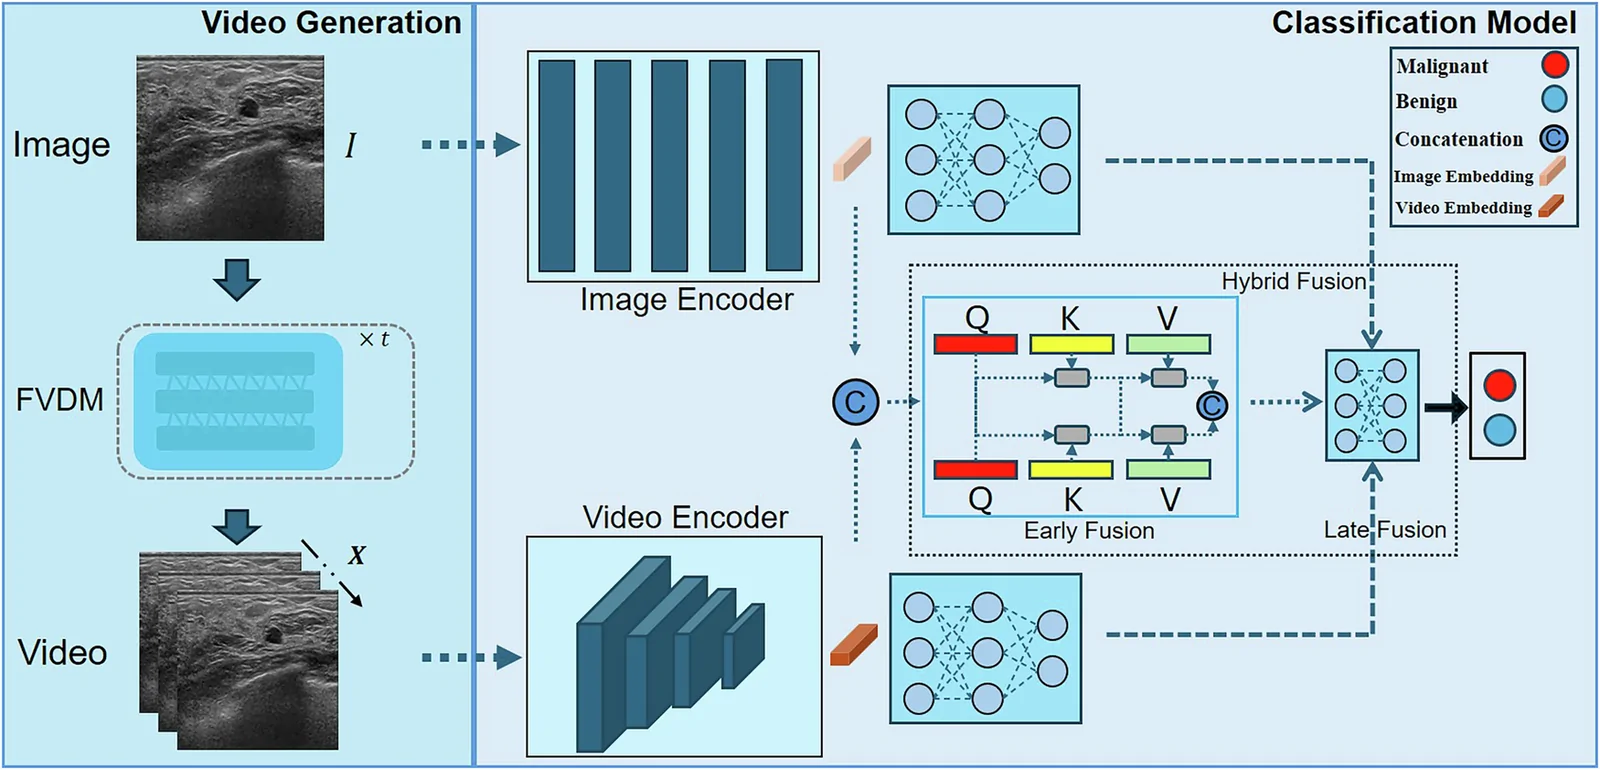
The proposed breast ultrasound classification framework consists of two stages. In the first stage, a diffusion-based model generates a breast ultrasound video from a static image. In the second stage, the generated video and the original static image are used to classify the breast tumor type.
